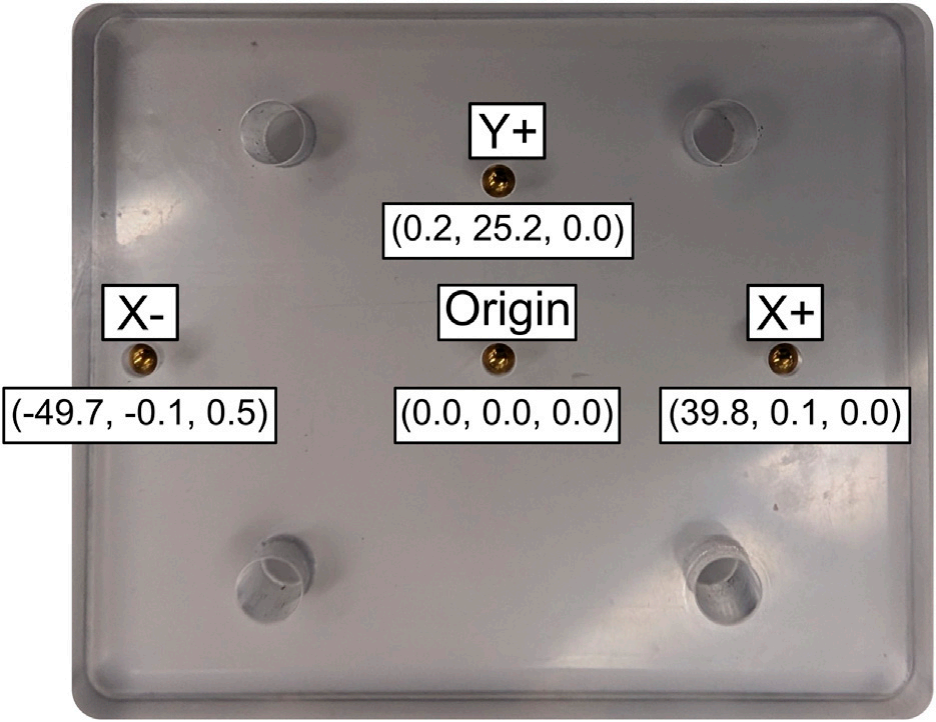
Precision-machined polycarbonate object with embedded beads. The CT-based reference bead locations are given in a local coordinate system (X, Y, Z) relative to the origin.
